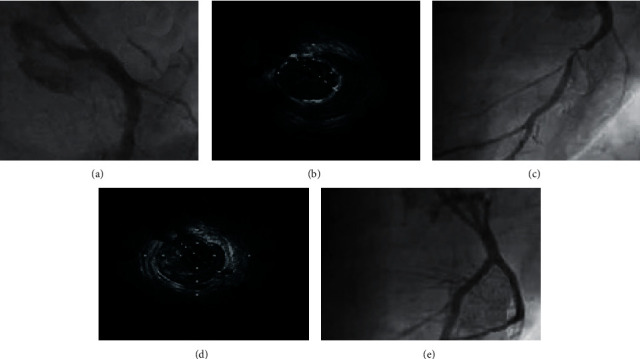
Intracoronary ultrasound imaging of a male patient before and after treatment (62 years old, admitted to the hospital for CHD unstable angina pectoris). A–C shows the intracoronary ultrasound imaging performance of the patient before treatment, the anterior descending branch was occluded with ischemia symptoms; D–E shows the intracoronary ultrasound imaging performance of the patient before and after treatment, occlusive coronary artery was successfully opened and the clinical effect was definite.
